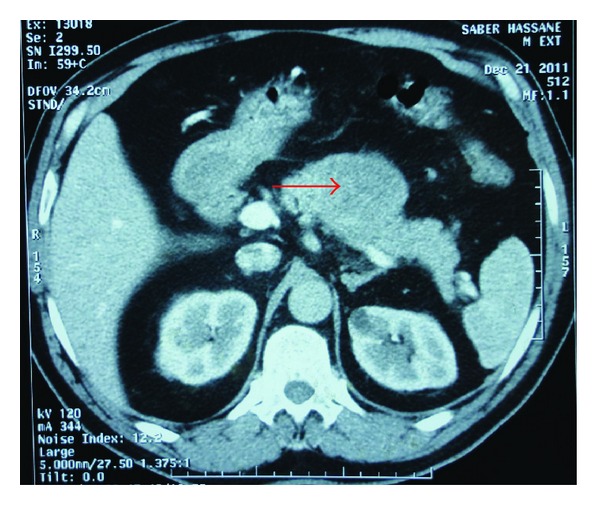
CT scan of the abdomen cut: showed a 50 mm mass in the pancreatic body, suggestive of metastasis from a primary in the lung.
